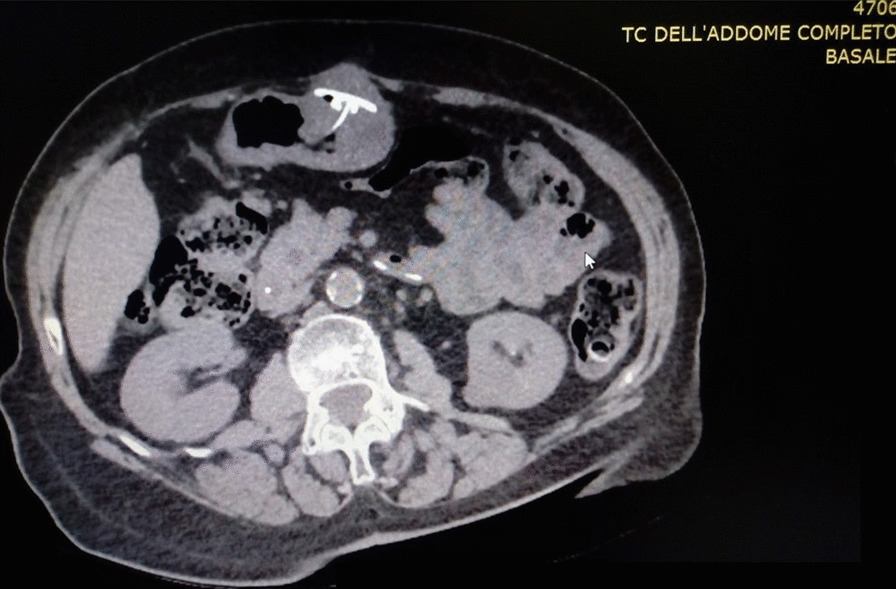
Computed Tomography (CT) image demonstrating a BBS type 3
